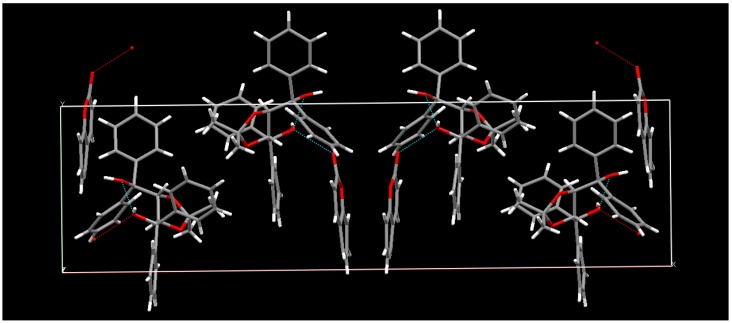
X-ray structure of the 1:1 inclusion complex of (−)-23 and 1.
